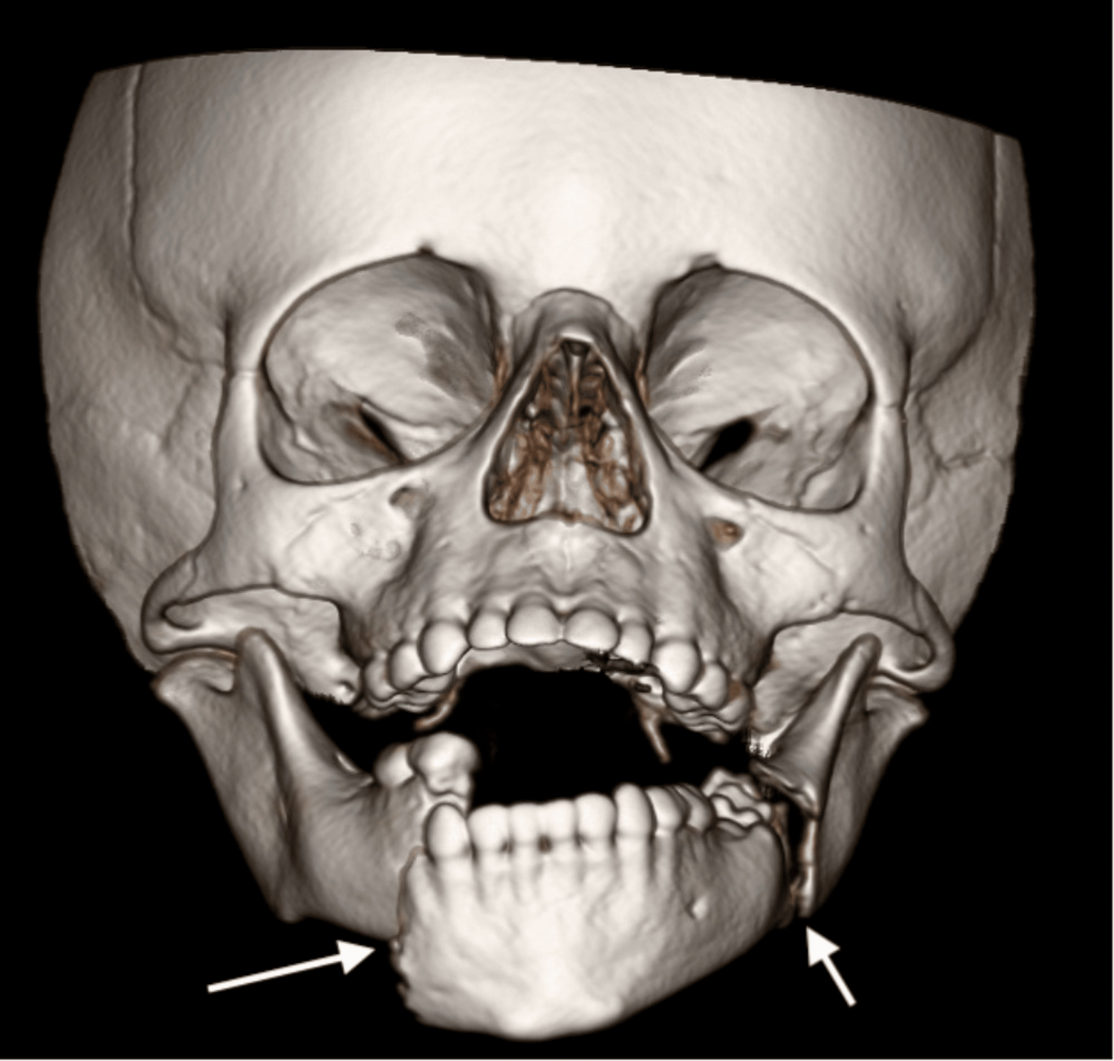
3D reconstruction of CT facial bones demonstrating acute bilateral mandibular fractures through the body of the right hemimandible and at the angle of the left hemimandible
